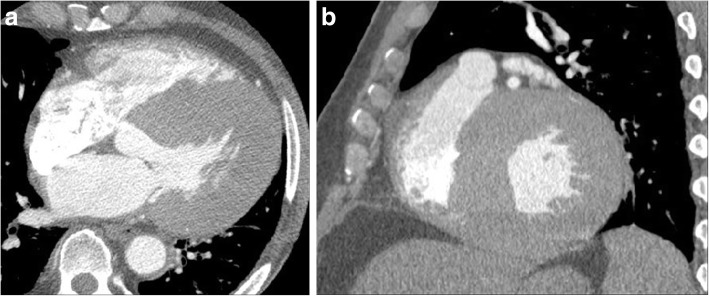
a Axial contrast-enhanced CT demonstrates left ventricular hypertrophy (LVH) on an axial contrast enhanced image in a 70-year-old man being evaluated for back pain. b Sagittal oblique multiplanar reformatted MPR images (short axis) demonstrate concentric hypertrophy of the left ventricular wall
